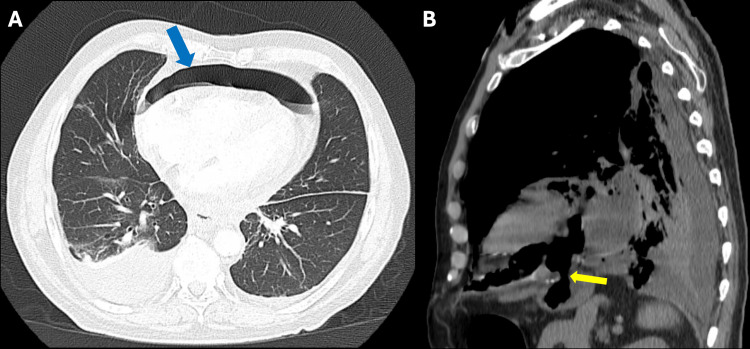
Chest CT scans from 2017 (A) CT scan on the day of presentation showing clear pneumopericardium (blue arrow). (B) CT scan taken two days later, following the patient’s acute onset of shoulder pain and shortness of breath. The yellow arrow indicates the location of the fistula.
